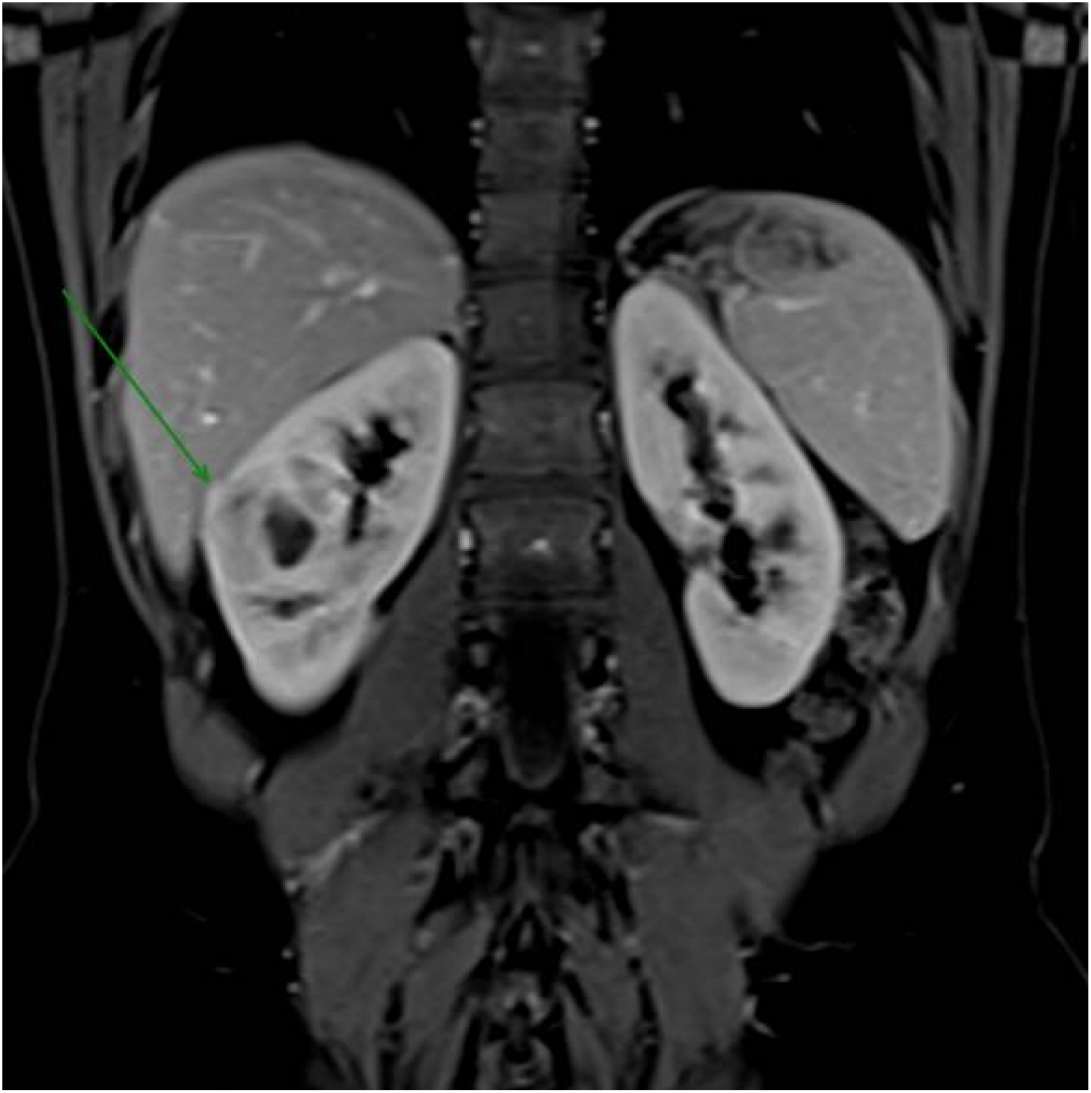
Magnetic resonance imaging revealed a right renal mass measuring approximately 44 mm in its largest dimension, increasing from 32 mm previously, characterizing a predominantly endophytic mass of indeterminate nature.
